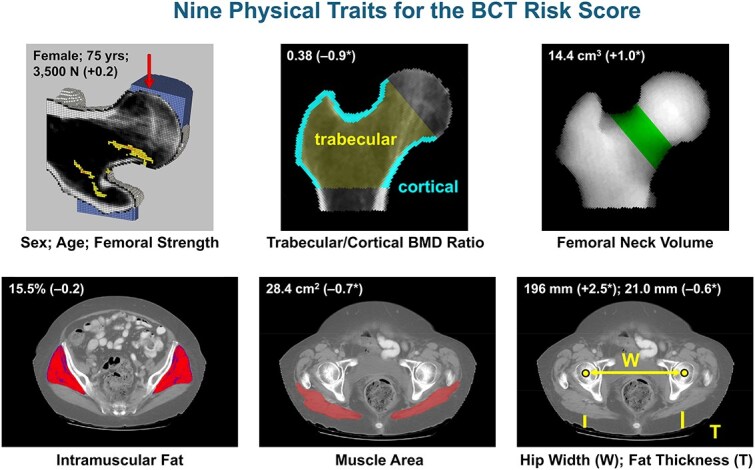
The nine physical traits used to calculate the BCT Risk Score. See Table 1 for a description of each physical trait. This particular patient was a 75-yr-old woman who had a BCT Risk Score of 91 (on a scale of 0-100). Her hip BMD T-score was −1.9 (osteopenia) and she fractured her hip 3 yr after the CT scan. Values of each measured physical trait for the patient are shown above, with Z-scores in parentheses; any starred value for a Z-score indicates that, compared to having an age- and sex-matched mean value of that trait (Z = 0.0), that trait is increasing her risk of hip fracture. Thus, in addition to her advanced age, this patient is at high risk of hip fracture because of low trabecular/cortical BMD ratio, large skeletal size (high FN volume, wide hips), small muscle size, and low fat thickness.
